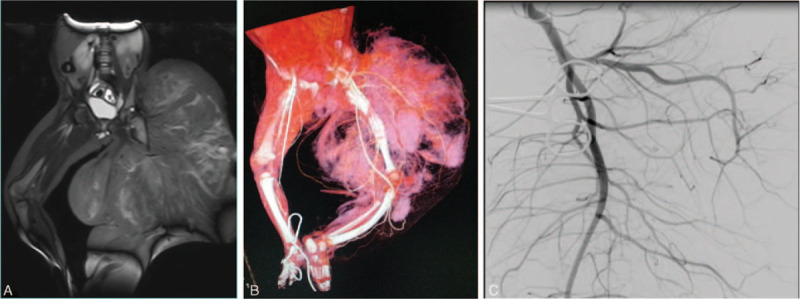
The preoperative MRI, CTA, and DSA examination of the patient. (A) The Axial and coronal MRI image demonstrated a giant mass with ill-defined border. The musculature, fascia, overlying subcuticular fat and skin infiltration was obvious. (B and C) CTA and DSA demonstrated increased and abnormal morphology of vascular branches.
